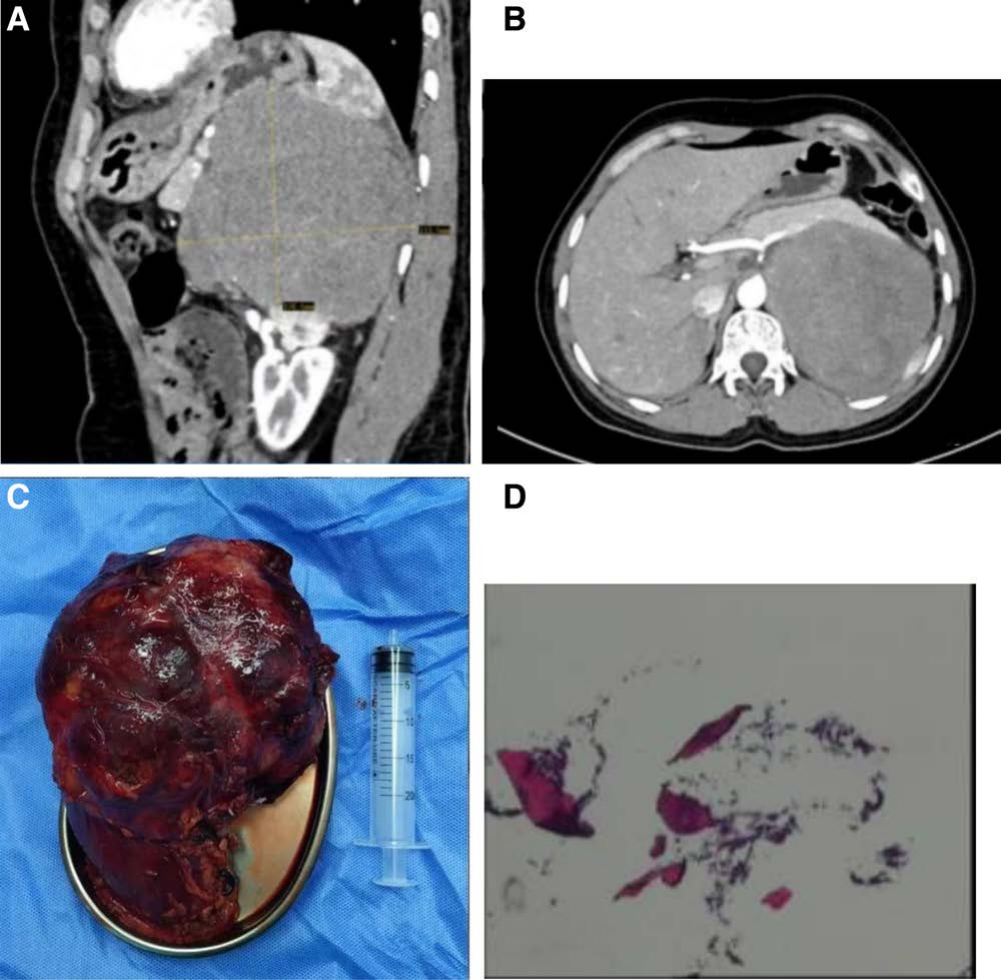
(A and B) Abdominal enhanced CT showed that the right adrenal nodule and the left adrenal region were huge space-occupying lesions, and pheochromocytoma was possible. (C) Postoperative adrenal tumor. (D) Pathological section after operation showed lymphoma.
